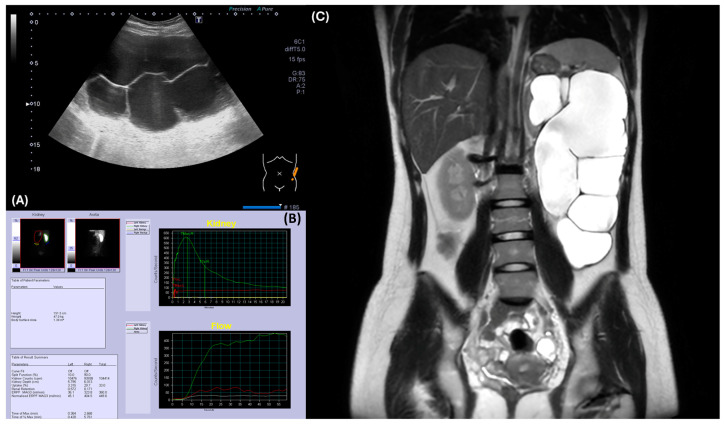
Radiographic evaluation of the left kidney revealed hydronephrosis and ureteropelvic junction obstruction. (A) Abdominal ultrasound demonstrated severe hydronephrosis in the left kidney. (B) Renal MAG-3 study indicated poor function of the left kidney, precluding assessment of obstruction. (C) MRI confirmed severe left hydronephrosis with a thinned renal cortex, suggesting ureteropelvic junction obstruction.
